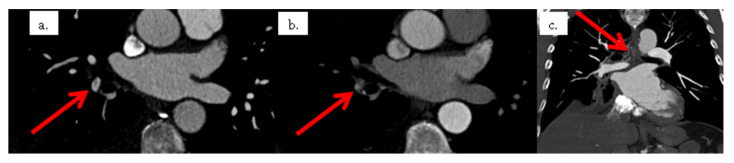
Second CT scan with two acquisition times. (a) shows axial early-acquisition CT with a segmental filling defect in a segmental artery of the right lower lobe. (b) shows axial late acquisition CT with, instead of a filling defect, the high enhancement of the same segmental pulmonary artery and of the bronchial arteries, while the other pulmonary arteries were not enhanced. (c) shows a coronal maximum intensity projection reformat of the late acquisition CT. It shows dilated systemic bronchial arteries feeding the right inferior lobar pulmonary neoplastic.
